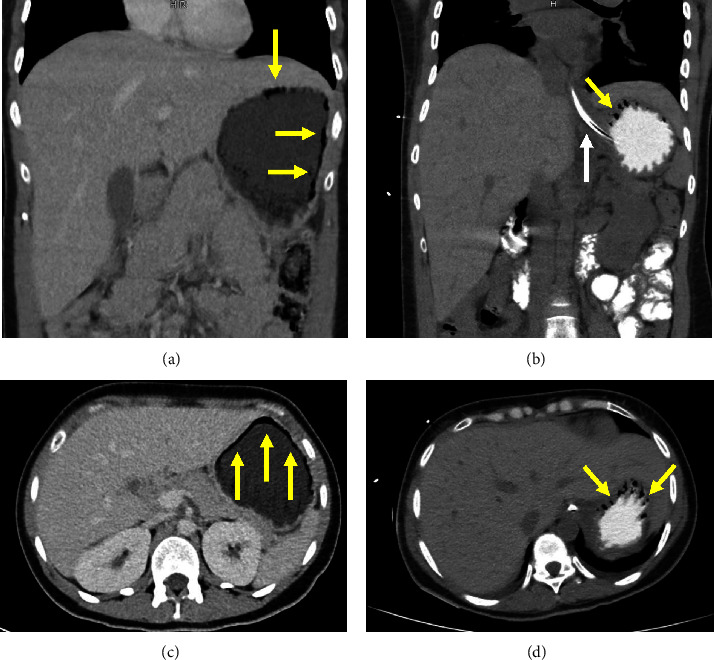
Coronal (a) and axial (b) images from the initial CT of the abdomen and pelvis with IV contrast demonstrate a distended stomach with pneumatosis (yellow arrows) within the gastric wall involving most of the stomach. No pneumoperitoneum, portal venous gas, or pneumatosis within the small or large bowel was identified. Coronal (c) and axial (d) images from CT of the abdomen and pelvis with IV and oral contrast taken on day four demonstrating oral contrast and an enteric tube (white arrow) within the stomach with near interval resolution of gastric pneumatosis. Residual pneumatosis in the wall of the anterior antrum (yellow arrows) is noted.
